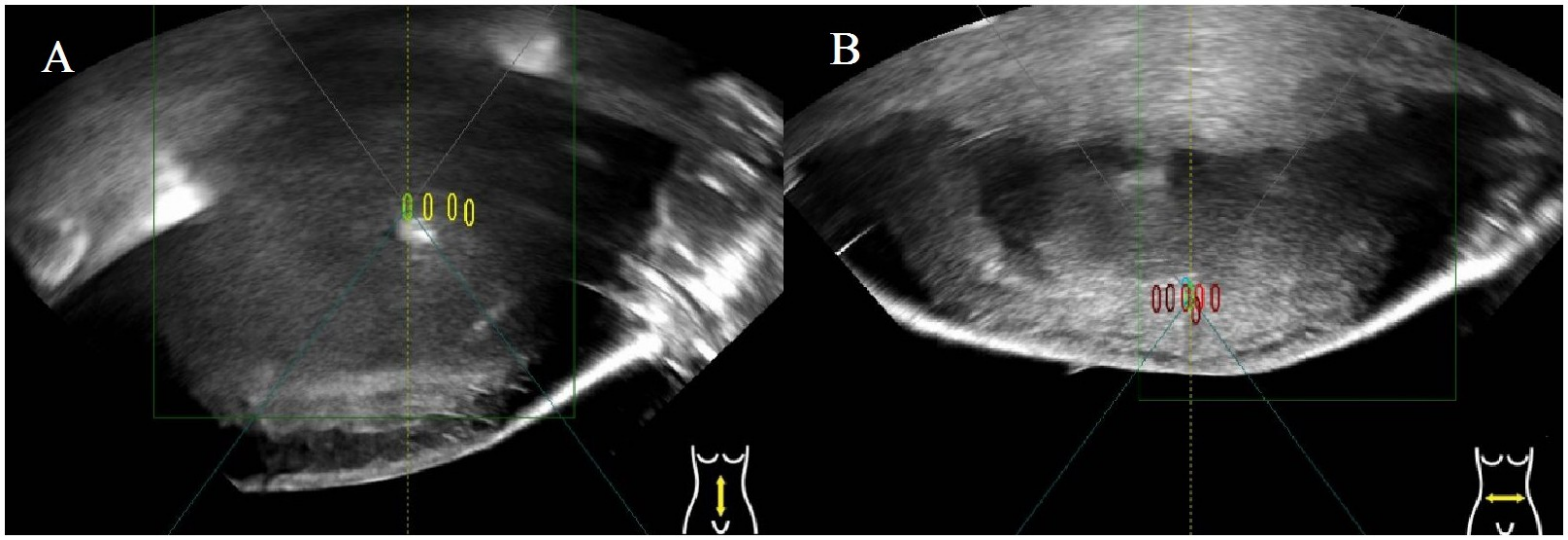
The real-time ultrasound image obtained from two patients with liver metastases. (A) shows the overall grayscale change: the gradient grayscale increases; (B) shows the Massive grayscale change: the area around the focal point quickly and noticeably grays and brightens grayscale We categorized grayscale changes into two types: overall grayscale changes and massive grayscale changes [1]. Overall grayscale change refers to a gradual increase in grayscale around the focal point, while massive grayscale change indicates an immediate and significant diffuse increase in grayscale in the focal area. However, there is no standard for grayscale changes in HIFU treatment for malignant tumors. Clinically, grayscale changes for malignant tumors are typically assessed based on the standard of grayscale changes in HIFU treatment for uterine fibroids.
